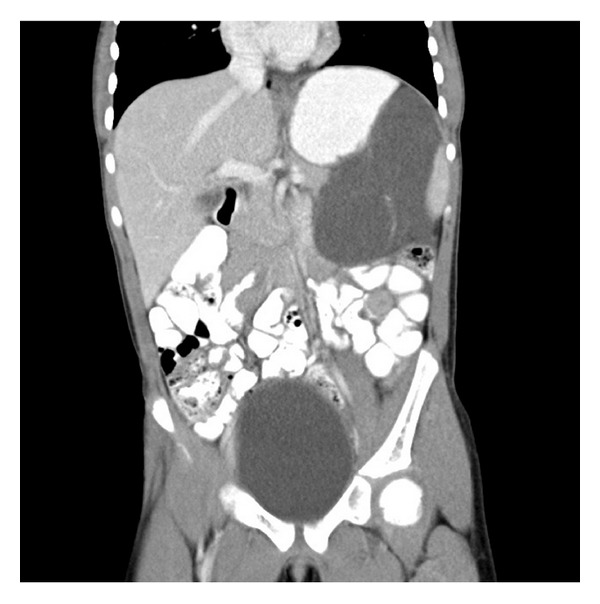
Contrast-enhanced CT coronal scan shows retroperitoneal lobulated-septated cystic mass that reaches to subdiaphragmatic space.
